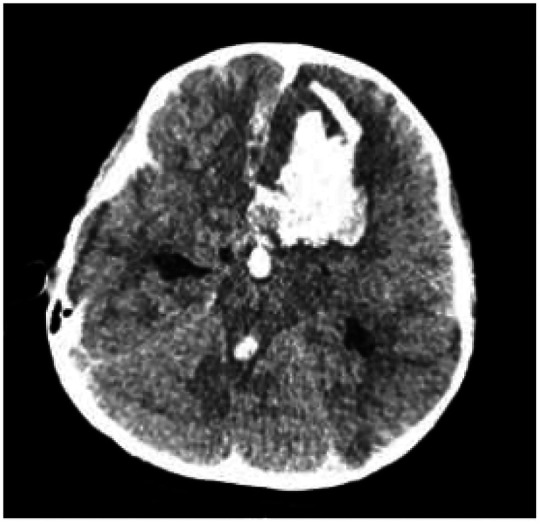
CT shows extensive intraventricular hemorrhage with marked signs of transtentorial herniation.
